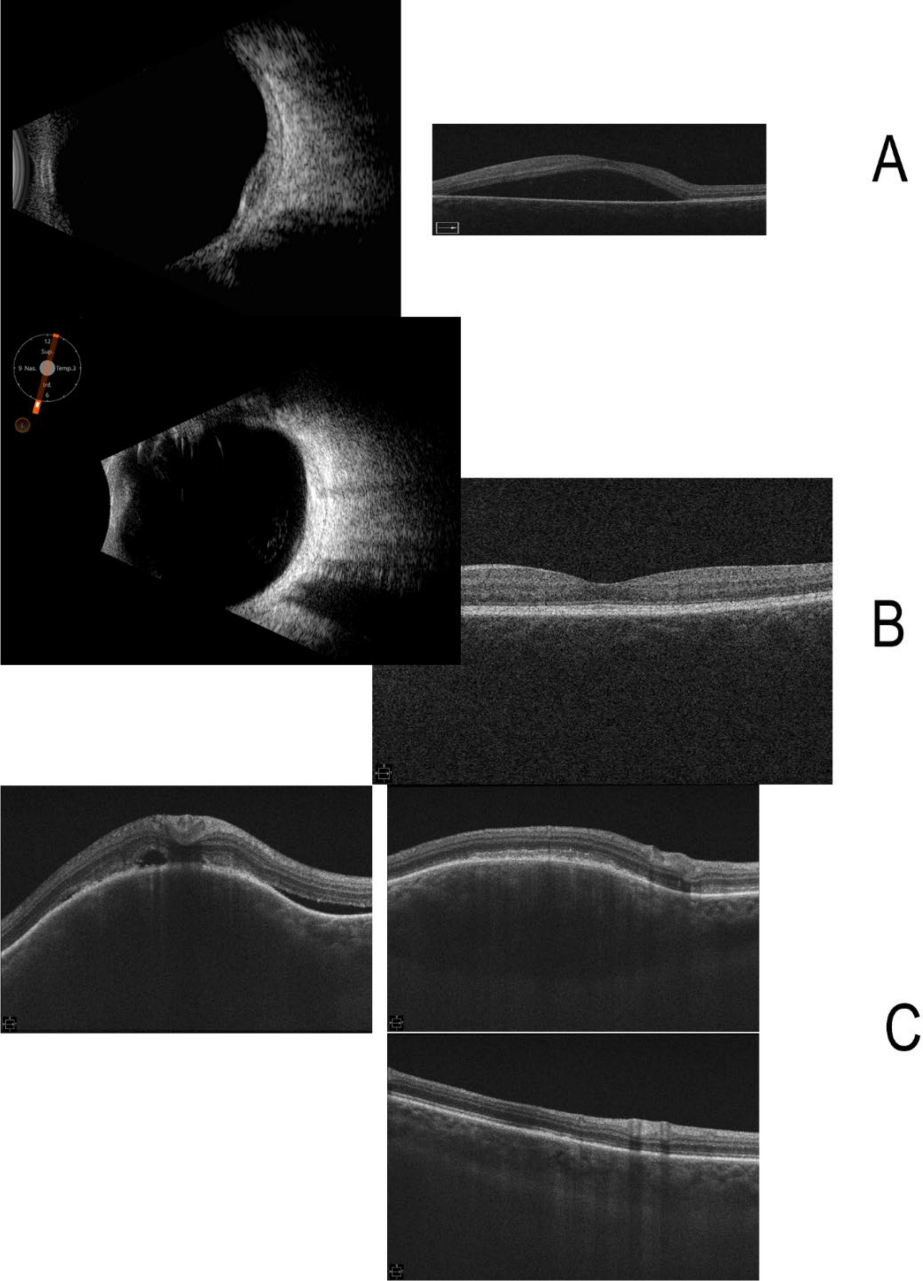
OCT and ophthalmic ultrasound images. (A) Initial B-scan ultrasound showing choroidal metastasis. (B) Last treatment timepoint -- B-scan ultrasound and macula. (C) Optical coherence tomography (OCT) showing cross-section through the choroidal metastasis and retina. The retinal detachment resorbed, and the choroidal metastasis showed good progressive regression.
